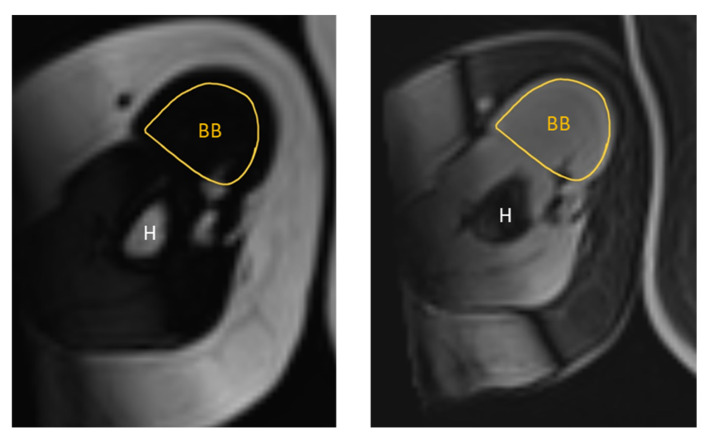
Representative T1-weighted Dixon VIBE MR image tracing the cross-sectional area of the right BB on the fat-based image (left) and water-based image (right). BB = biceps brachii, H = humerus.
